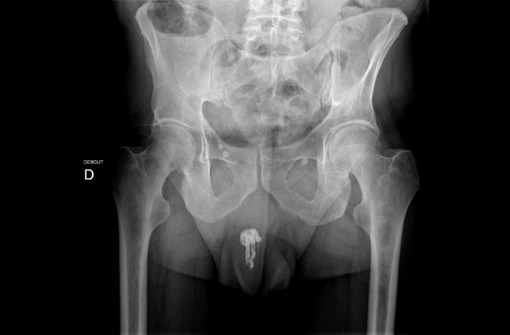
Aspect du corps étranger à la radiographie standard du bassin
